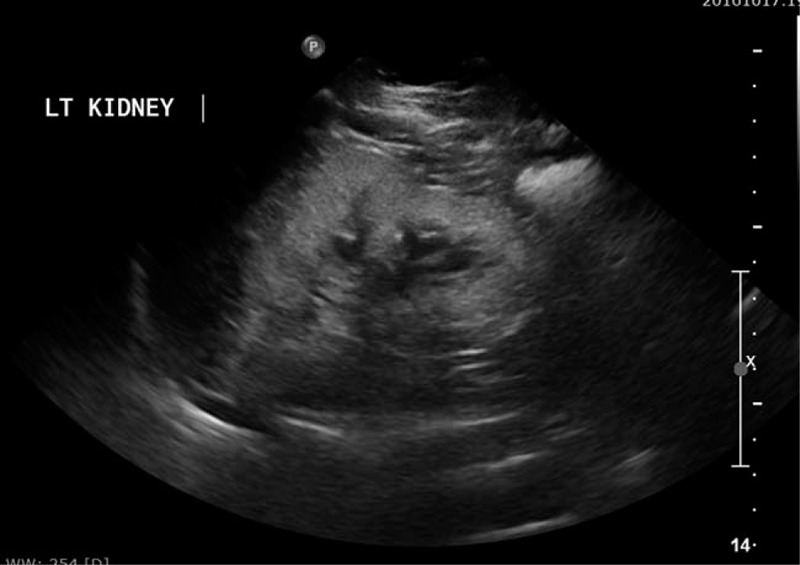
Ultrasound of left kidney obtained in sagittal view demonstrates increased echogenicity.
